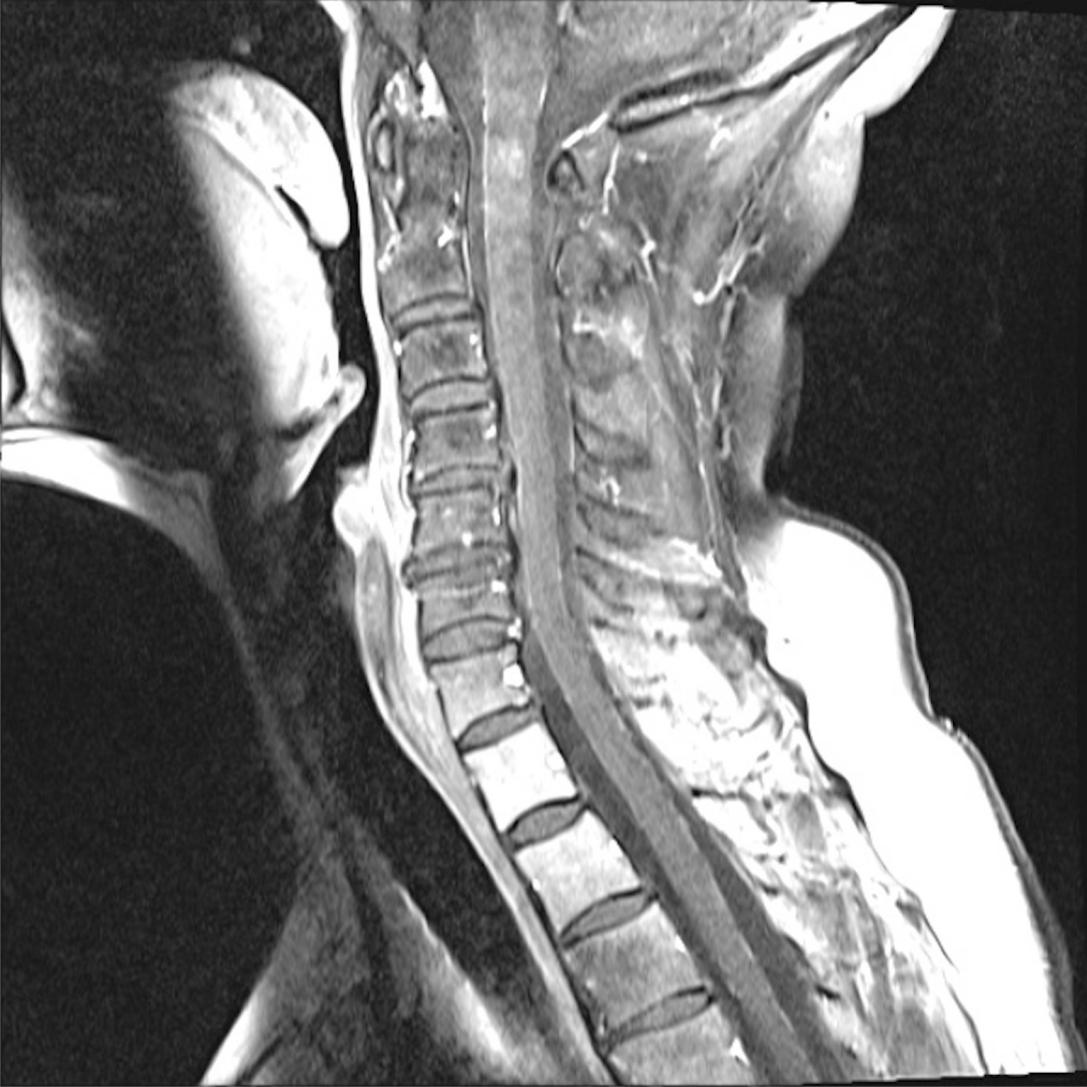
Cervical Spine MRI Sagittal MRI of the cervical spine demonstrated nodular contrast enhancement of the lesion at the cervicomedullary junction.
